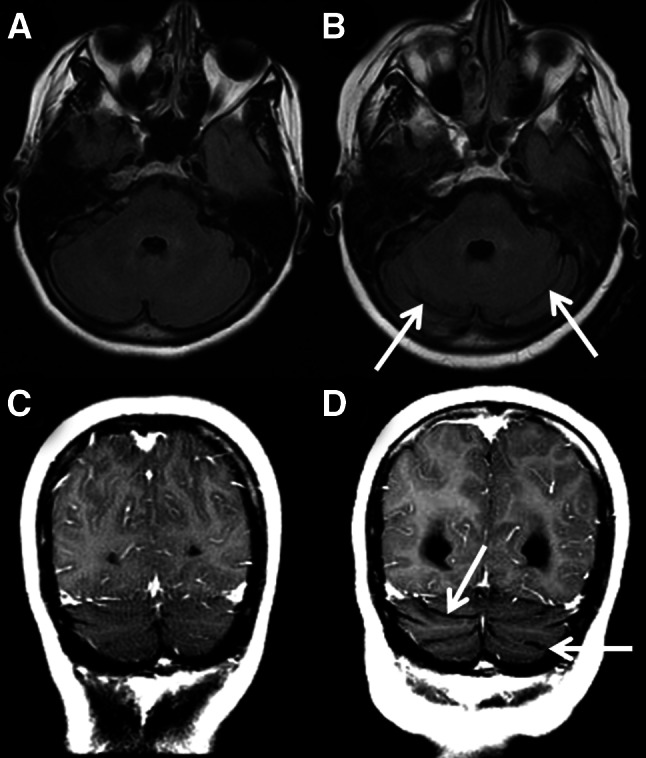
Axial fluid attenuated inversion recovery (FLAIR) images (a, c) and coronal T1 post-contrast images (b, d) of patient 2 showing no cerebellar atrophy at the time of PML diagnosis (grade 0, a, b) and grade 1 cerebellar atrophy 2.5 months later (c, d). Note the bilateral dilated sulci in image c and d (*open arrowheads*)
